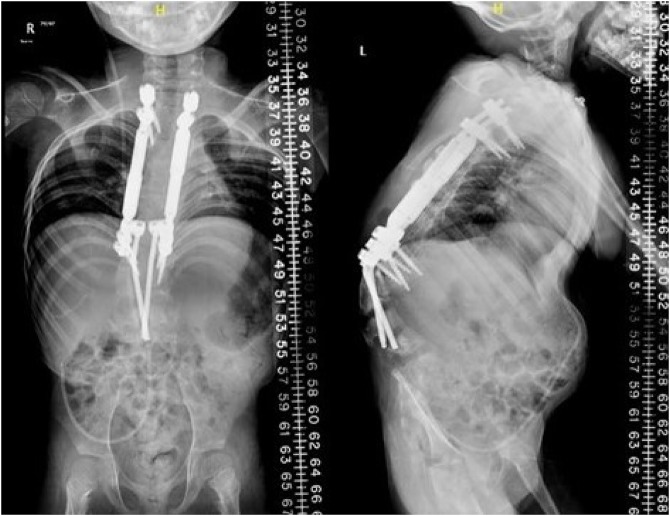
Subsequent AP and lateral radiographs after fixation with magnetically controlled growing rod expansions. The rod has cut-out at the superior aspect of the L5 body. This was due to the lumbar spine *growing off* the rods distally, which is one of the expected outcomes (and benefits) of this technique.
